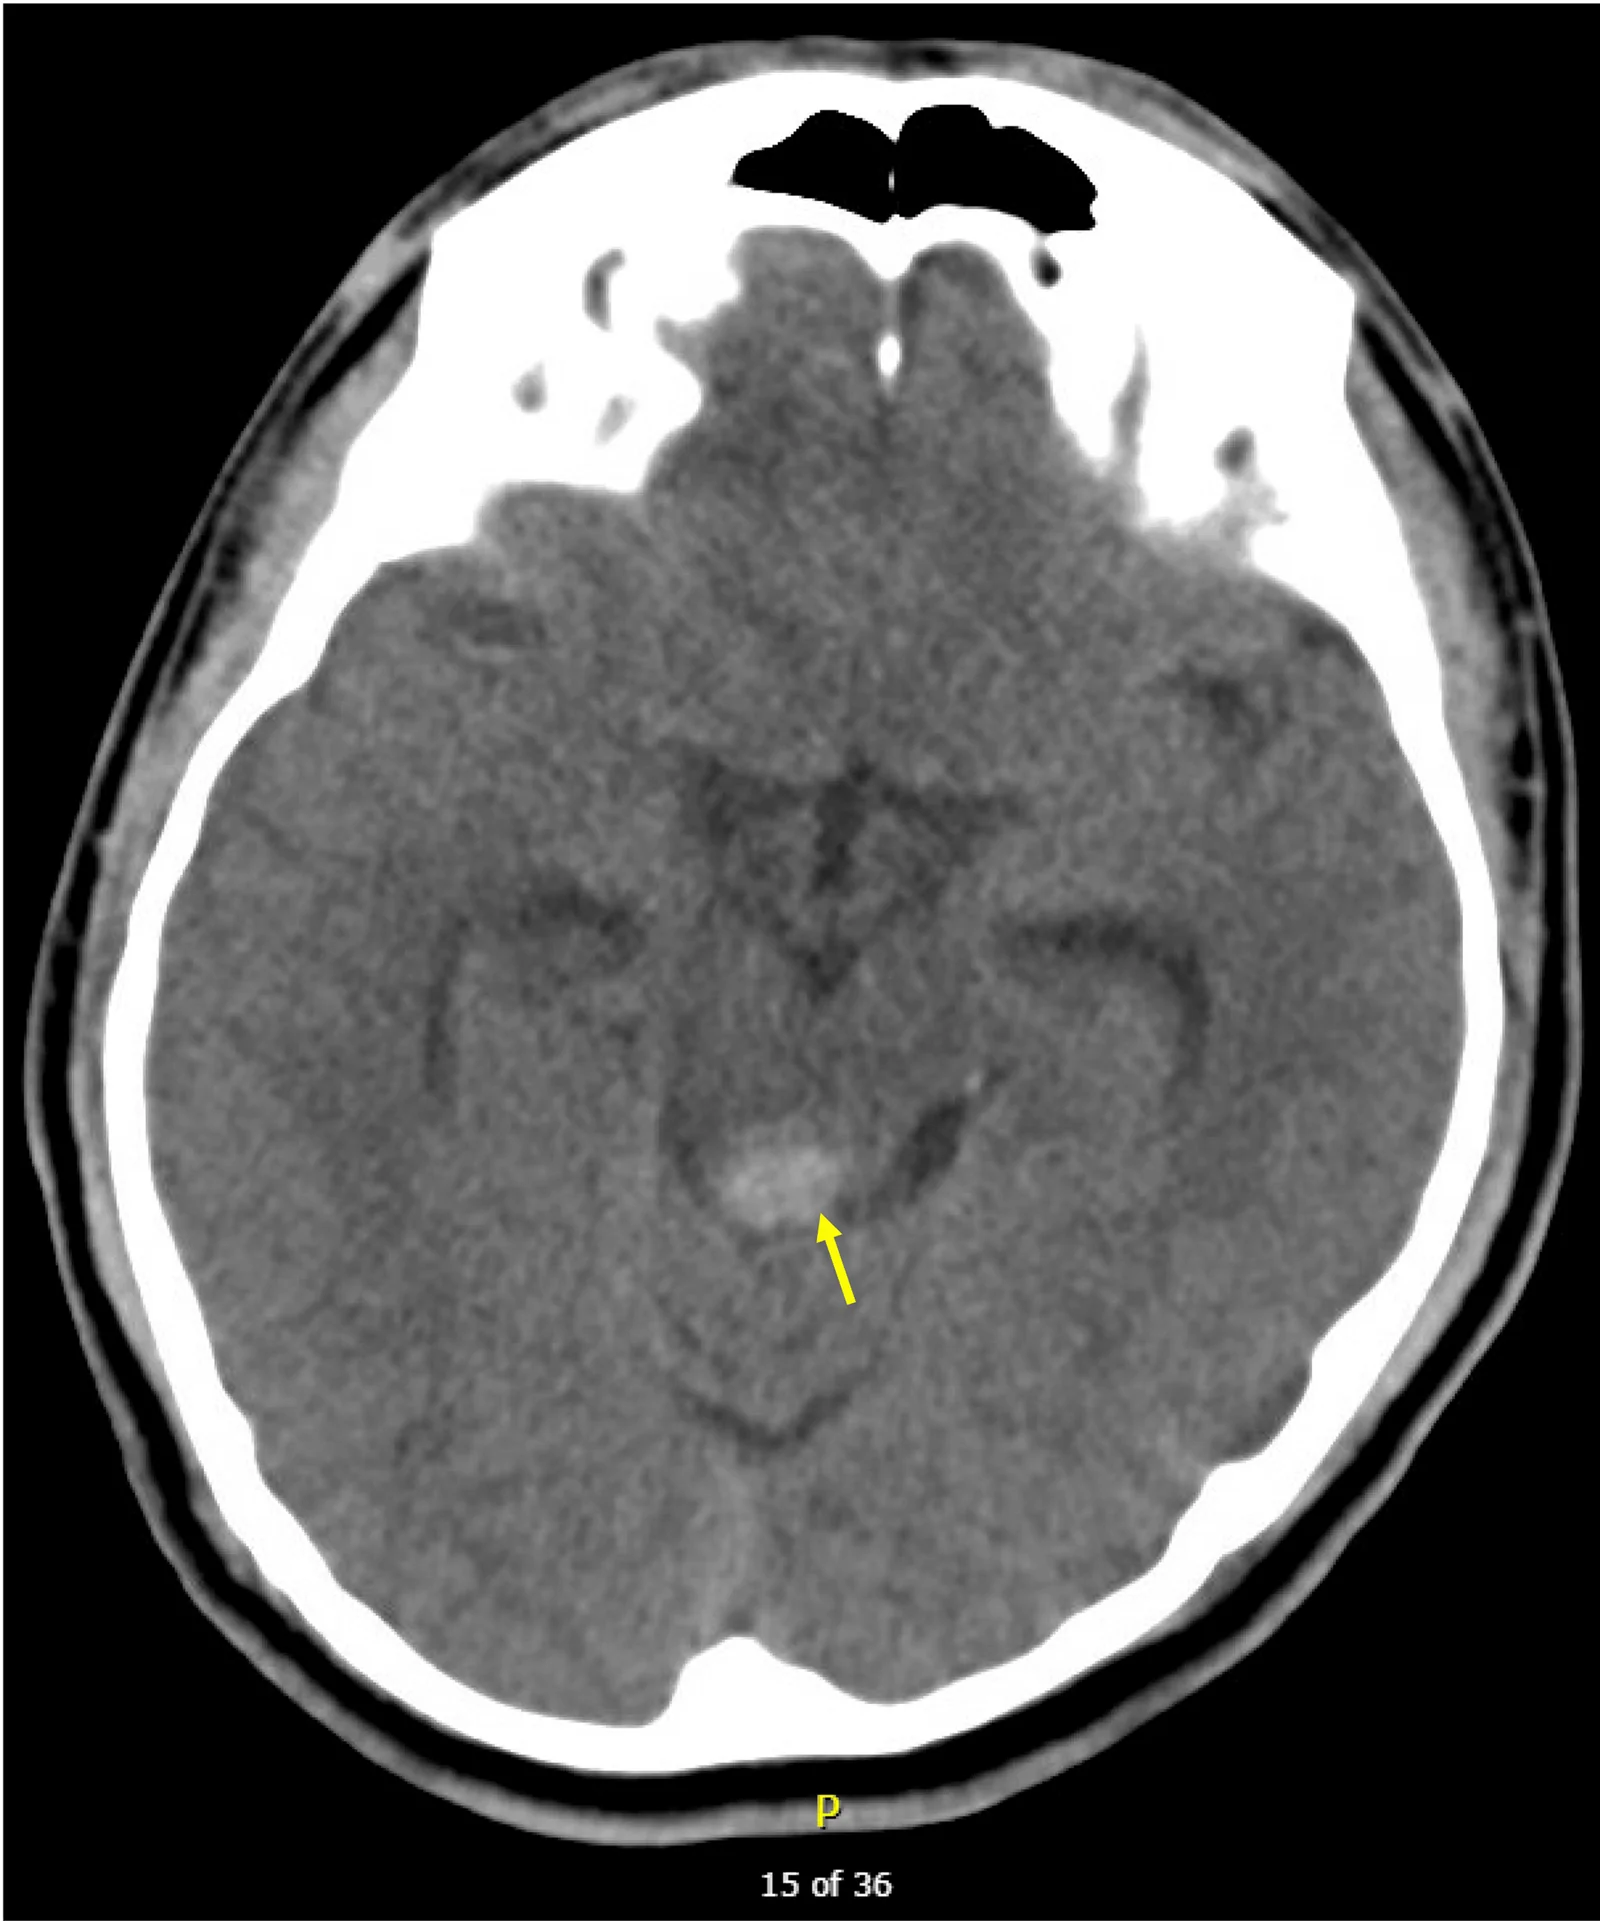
Non-contrast computed tomography (CT) of the brain demonstrating new subarachnoid hemorrhage (SAH) Representative axial slice from the index presentation. Arrow points to the region of the inferior colliculi within the quadrigeminal cistern.
